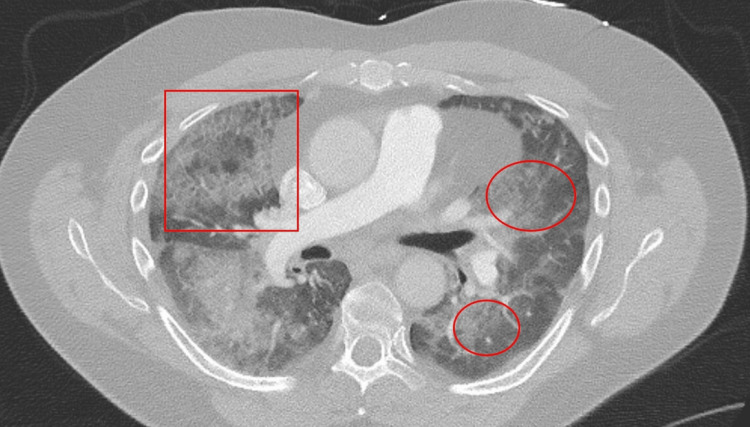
Computed tomography angiography (CTA) of the chest demonstrating a crazy-paving pattern (box) with superimposed ground-glass opacities (circles).
